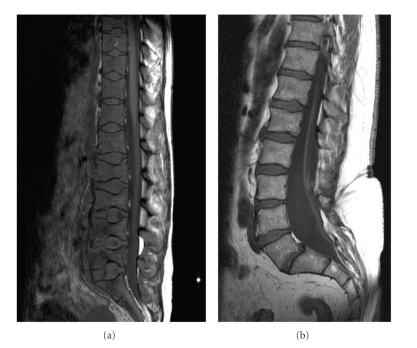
(a) shows a T1W sagittal MRI spine of a patient with sickle cell anaemia with diffuse low signal from the vertebral bodies consistent with hyperplastic haematopoietic red marrow replacing the normal bright fatty marrow. Note smooth concavity of the vertebral endplates at multiple levels from bone softening (fish mouth vertebra). Normal appearance of marrow is depicted on (b) for comparison.
